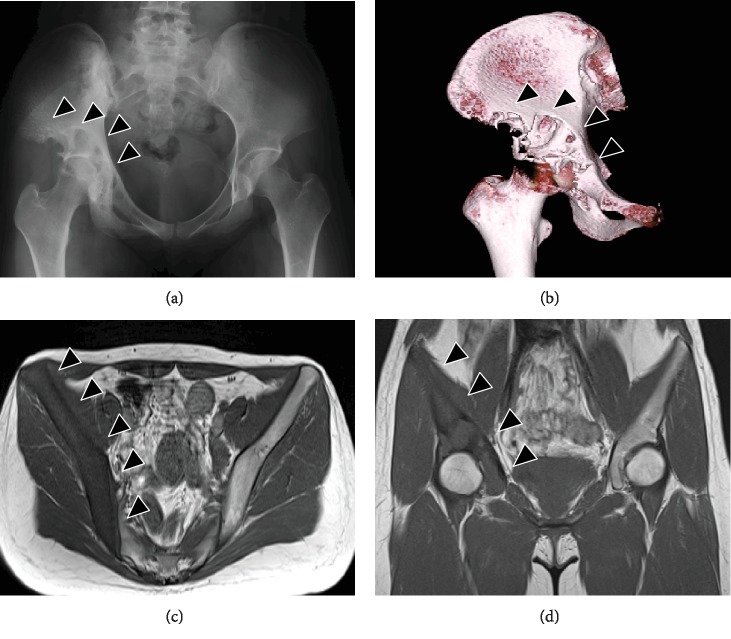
(a, b) Radiography and three-dimensional computed tomography (3D-CT) reconstruction showed an osteolytic lesion of the right ilium and acetabulum with cortical destruction, marginal sclerosis, slight pseudotrabeculation, and bone expansion (arrowhead). (a) Radiograph. (b) 3D-CT. (c, d) Magnetic resonance imaging revealed a massive tumor lesion throughout the right ilium; T2-weighted images of the ilium lesion showed low-signal intensity compared to muscle (arrowhead). (c) Axial view. (d) Coronal view.
